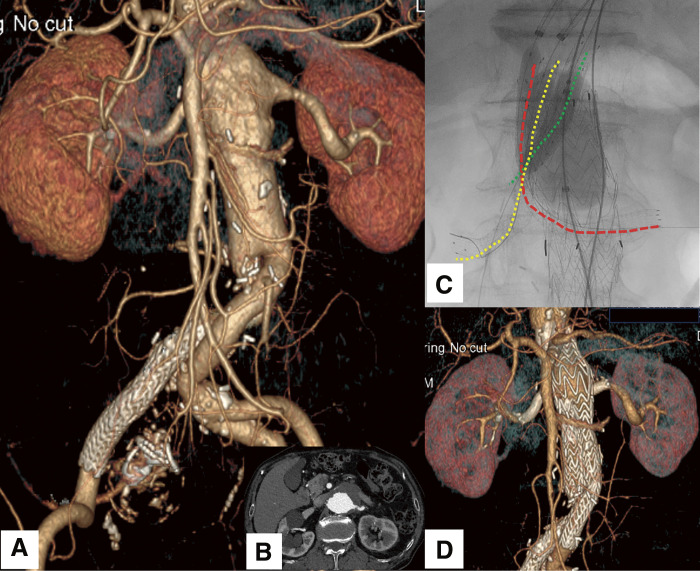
Fig. 1 (A) CTA reveals a pararenal AAA involving both renal arteries. (B) Axial imaging demonstrates a pararenal AAA. (C) During ch-EVAR, simultaneous kissing balloon technique is employed to minimize gutter-related endoleaks. The red dotted line delineates the displaced left renal artery chimney stent graft protruding into the aneurysm sac with an oblique trajectory. The yellow dotted line demarcates the right renal artery, while the green dotted line represents the SMA chimney stent graft. (D) At 6 months postoperatively, CTA confirms maintained patency of the chimney stent grafts and the absence of detectable endoleaks. ch-EVAR: chimney endovascular aneurysm repair; AAA: abdominal aortic aneurysm; CTA: computed tomography angiography; SMA: superior mesenteric artery
